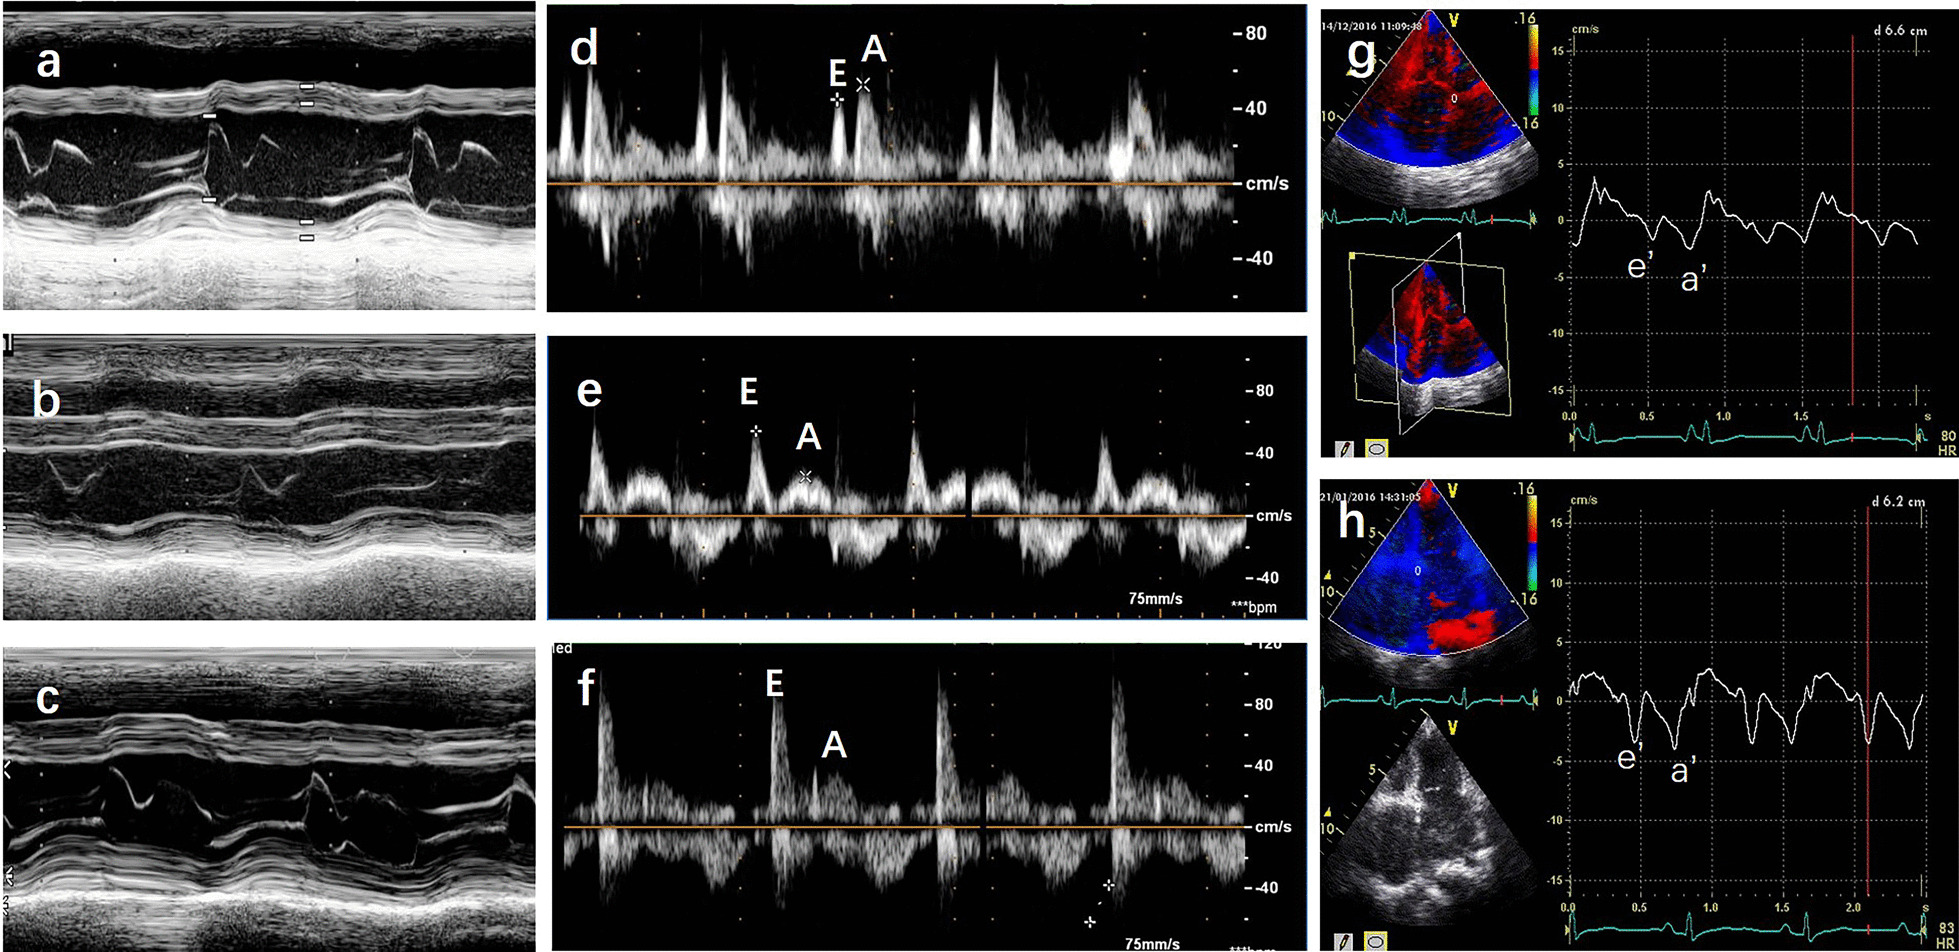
Representative images of M-mode echocardiography and diastolic function of left ventricles. E: peak mitral flow velocity in early diastole; A: peak mitral flow velocity in late diastole. IVRT: isovolumic relaxation time. E′: peak velocity of mitral annulus in early diastole; a′: peak velocity of mitral annulus in late diastole. a (case 1), b (case 2), c (case 4): M-mode echocardiography showed no abrupt septal movement (‘notch’ or ‘bounce’ in early diastole, or septal movement toward left ventricle in inspiration). d (case 1), e (case 2), f (case 4): Mitral flow velocity by pulsed Doppler echocardiography showing reduced cardiac diastolic function. g (case 1), h (case 4): Tissue velocity imaging of mitral annulus showing reduced cardiac diastolic function
